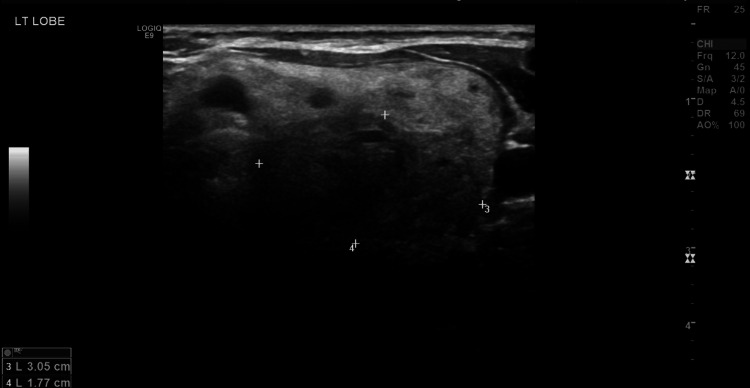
Follow-up ultrasound showing enlargement of the ill-defined left lobe nodule
